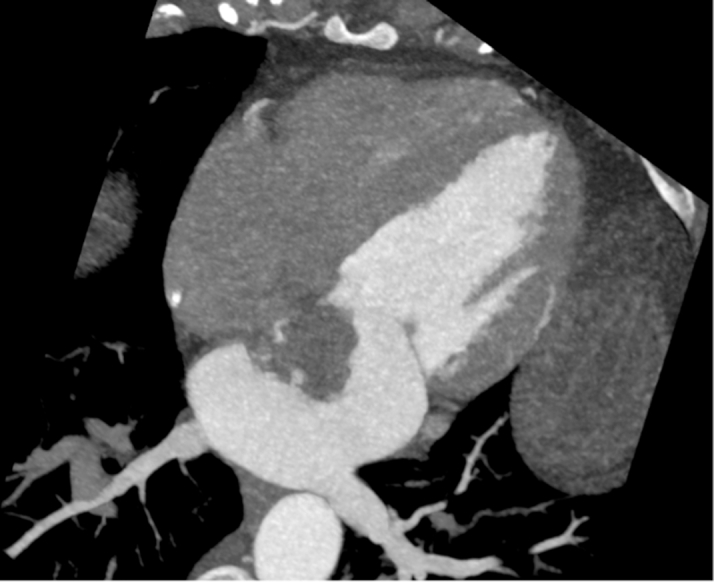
Cardiac Computed Tomography Left atrial myxoma with attachment to the interatrial septum; ovoid with lobulated surface, broad base, appears as an intracavity filling defect in the left atrium; no evidence of calcification.
